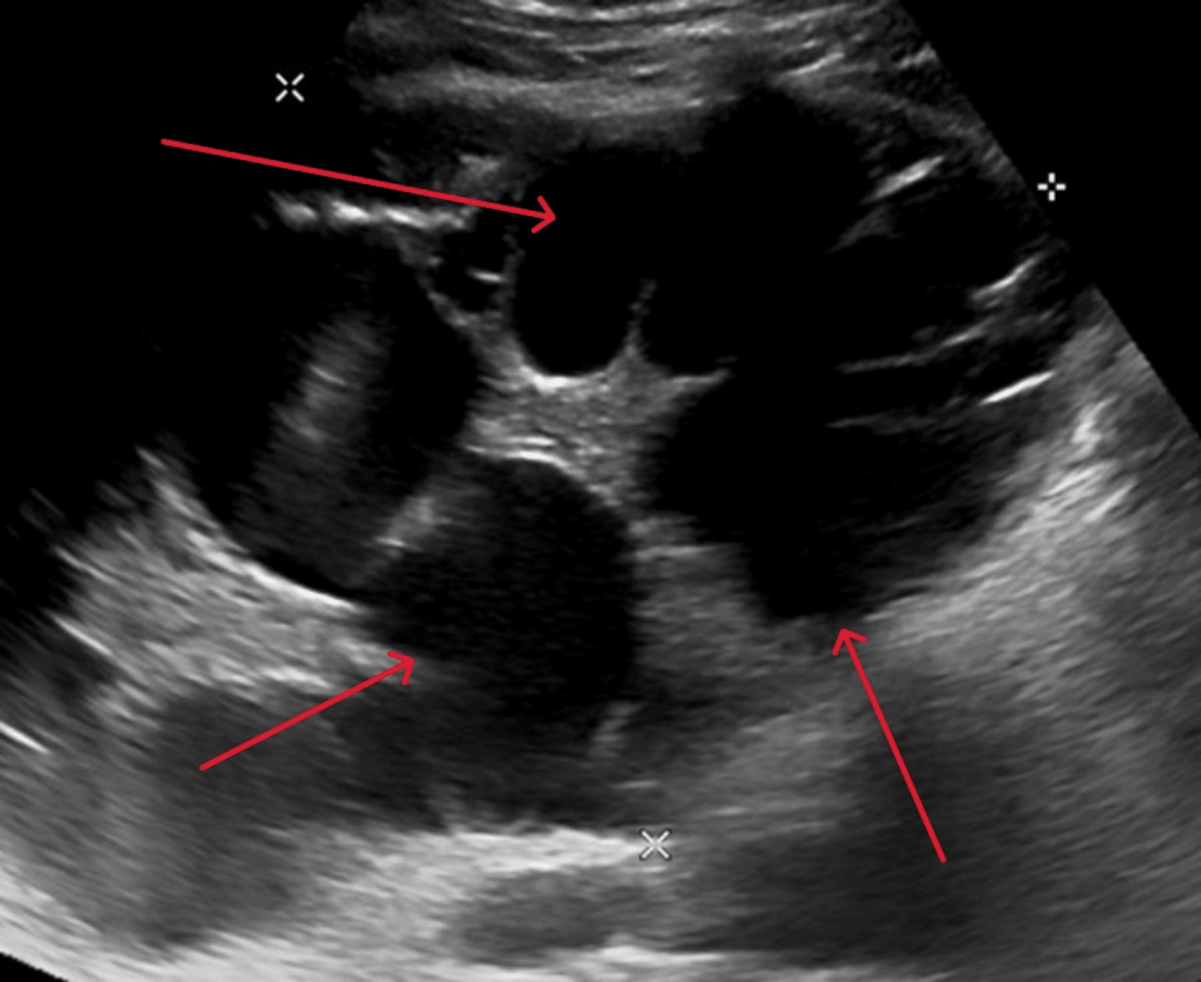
Multiloculated cystic lesion in the right lobe of the liver Abdominal ultrasound showing mild hepatomegaly (liver span: 15.5 cm) and a large multiloculated cystic lesion measuring 16 × 12 cm located in the right hepatic lobe (highlighted by red arrows).
